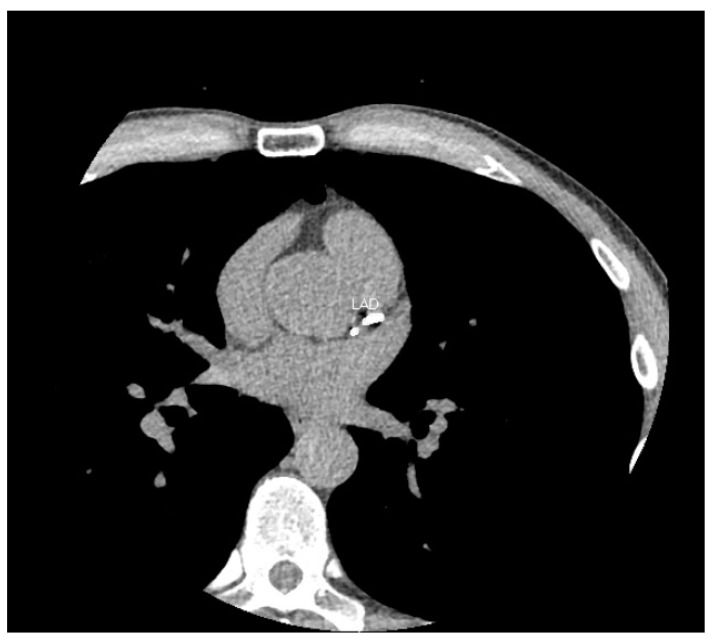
CT calcium score scan. Here is an axial image from a CT calcium score scan of a 51-year-old male. At his age, the majority of males do not exhibit coronary calcification. His total score > 400 places him at greater than the 97th centile for age and gender. The left anterior descending (LAD) artery is labelled.
